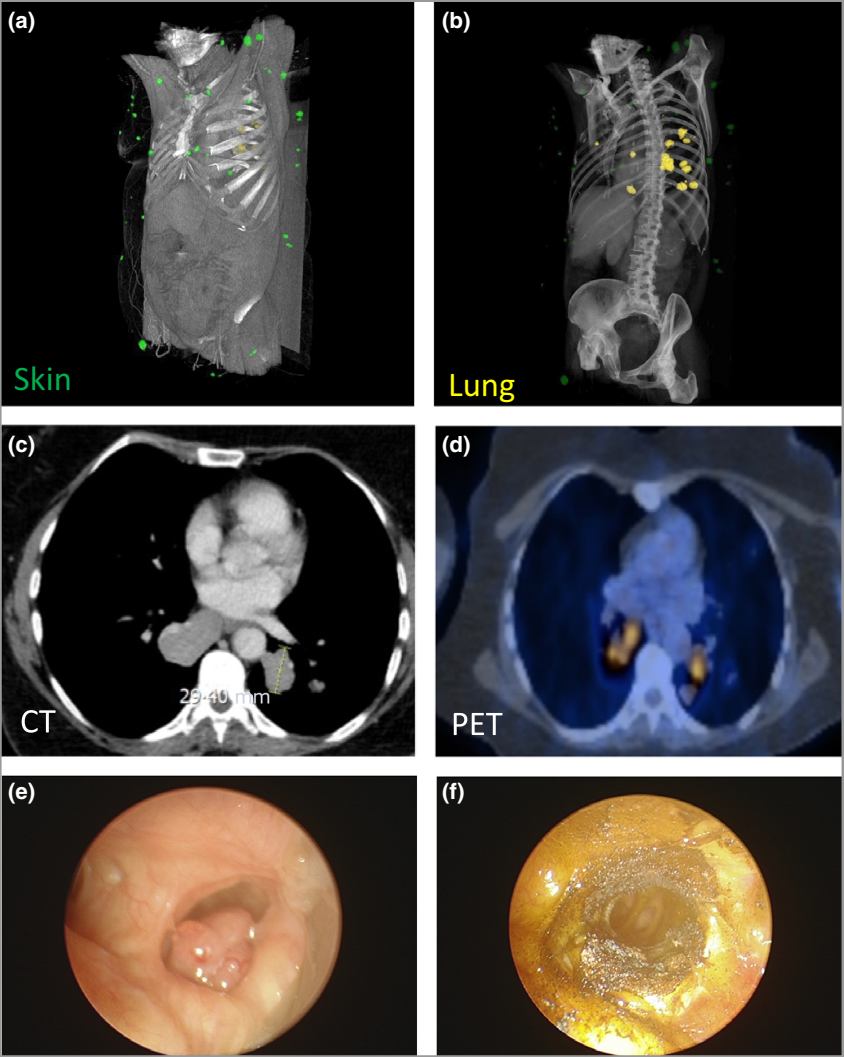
Clinical characterization of pulmonary cylindromas. (a) Three‐dimensional reconstruction and visualization of high‐resolution computed tomography (CT) images demonstrate the presence and morphology of cutaneous cylindromas (green lesions) and (b) pulmonary cylindromas (yellow lesions). (c) High‐resolution CT image of pulmonary cylindroma measuring 29·4 mm. (d) Fludeoxyglucose (FDG) positron emission tomography (PET) demonstrating high uptake in pulmonary cylindromas. Endobronchial views of pulmonary cylindroma (e) before and (f) after Nd‐YAG (neodymium‐doped yttrium aluminum garnet) laser ablation to restore airway patency.
